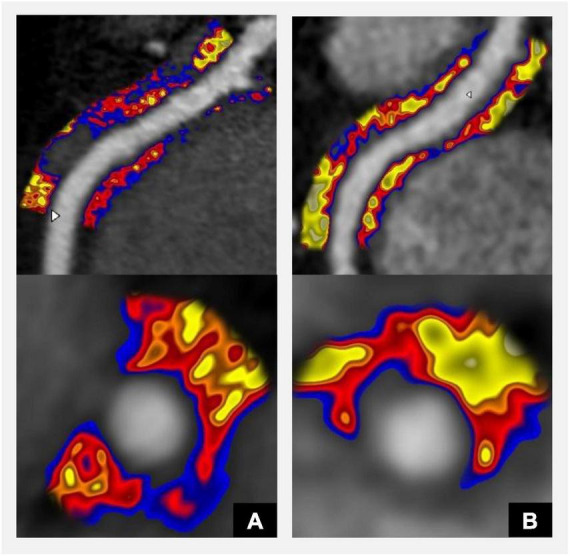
CT coronary angiography derived Fat Attenuation Index (FAI) derived from the analysis of Perivascular Adipose Tissue (PVAT) surrounding coronary arteries detects inflammation and may be used to guide the management of patients with diabetes and increased cardiovascular risk. Red-blue colour indicates inflammation and correlates with high risk of future cardiovascular events; yellow colour indicates low inflammation (55). FAI score can be provided for each of the main coronary arteries and considers FAI weighted for tube voltage, anatomical factors, and basic demographics. (A) Significantly increased coronary artery inflammation (FAI score 95th centile). (B) Follow-up scan after initiation of statin treatment shows significant reduction in inflammation (FAI score 58th centile). Panels (A,B) show CPR (top panel) and axial views (bottom panel).
